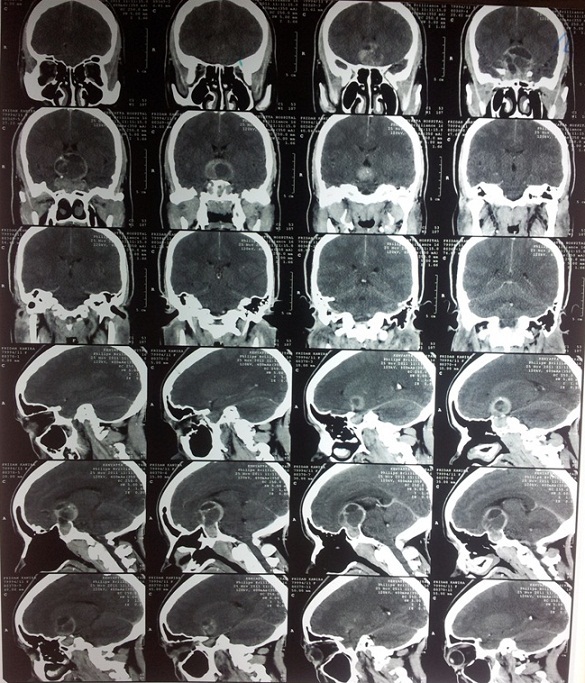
Axial slices of the patient's CT scan with contrast showing a sellar-suprasellar tumor which is cystic and has capsular enhancement
